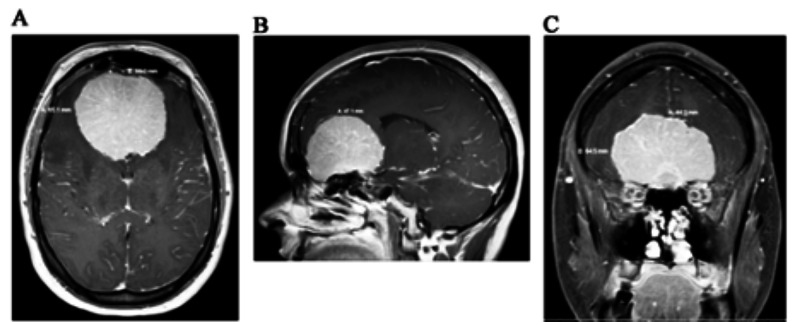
Pre-Op MRI MRI of the brain with contrast enhanced T1 (A) axial, (B) saggital, and (C) coronal reveals an enhancing 6.0 x 6.5 x 4.7 cm homogenous enhanced extra-axial mass consistent a giant olfactory groove meningioma.
